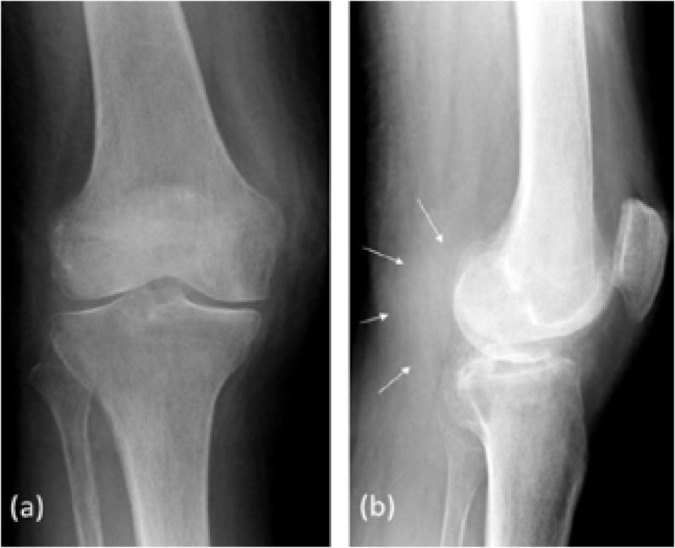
RIGHT knee (a) Frontal and (b) Lateral X-ray at initial presentation. Osteopenic bony appearances. Small volume suprapatellar joint effusion. Small popliteal fossa soft tissue swelling (arrows). Mild narrowing of joint spaces with no obvious osteophytes. No erosive bony changes.
